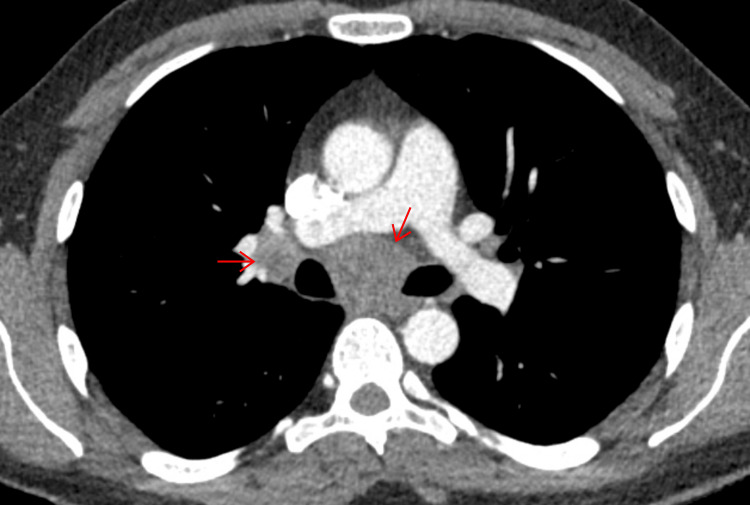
CT thorax showing enlarged subcarinal and right hilar lymphadenopathy CT: computed tomography
